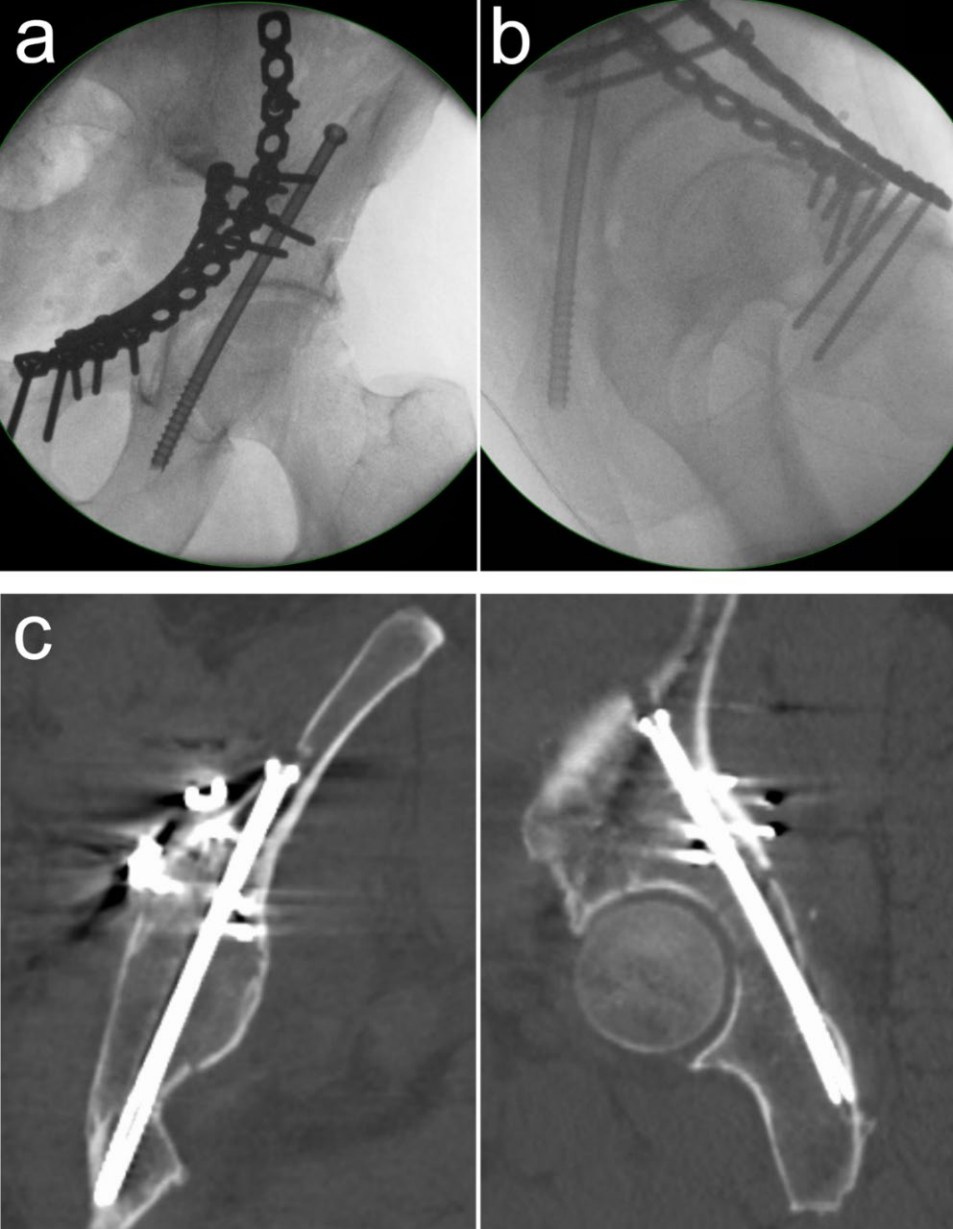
Correct screw placement a Intraoperative ap view of an ACPHT fracture treated with open reduction and plate fixation of the anterior column and PCS for the fixation of the posterior hemitransverse fracture. The ap view shows the correct screw trajectory in the coronal plane. b The lateral oblique view shows the correct screw trajectory in the sagittal plane as well as an extraarticular screw trajectory with no perforation of the posterior cortex of the posterior column. c The postoperative CT scan confirms the intraoperative findings. The posterior hemitransverse fracture is anatomically reduced. Coronal reconstruction (left) and sagittal reconstruction (right)
